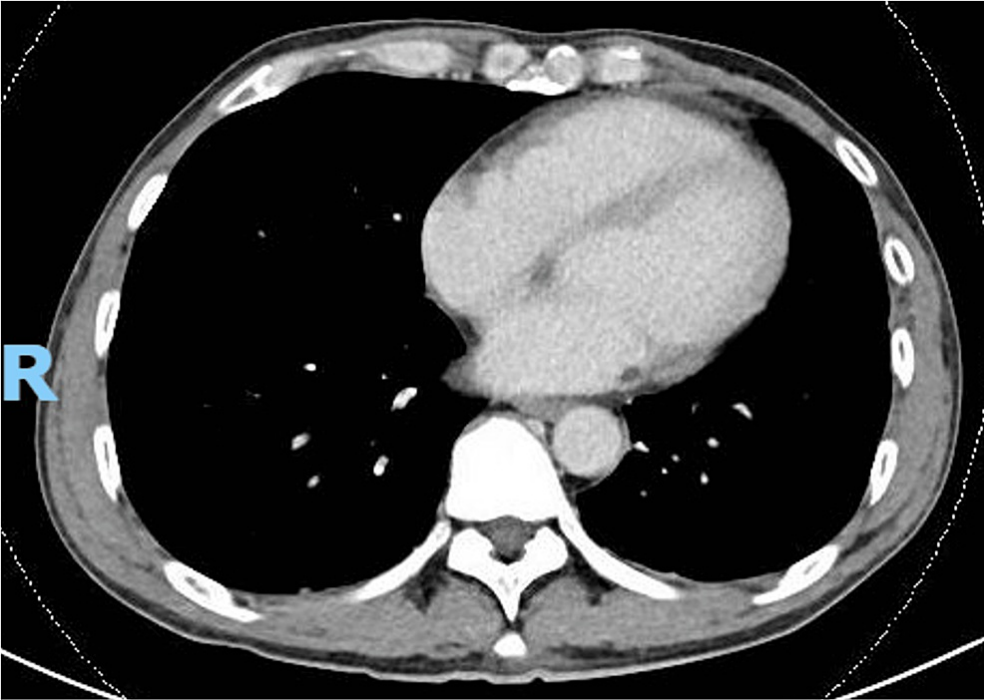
Computed tomography of the thorax showing resolution of the subpleural lesion in the right lower lobe.
